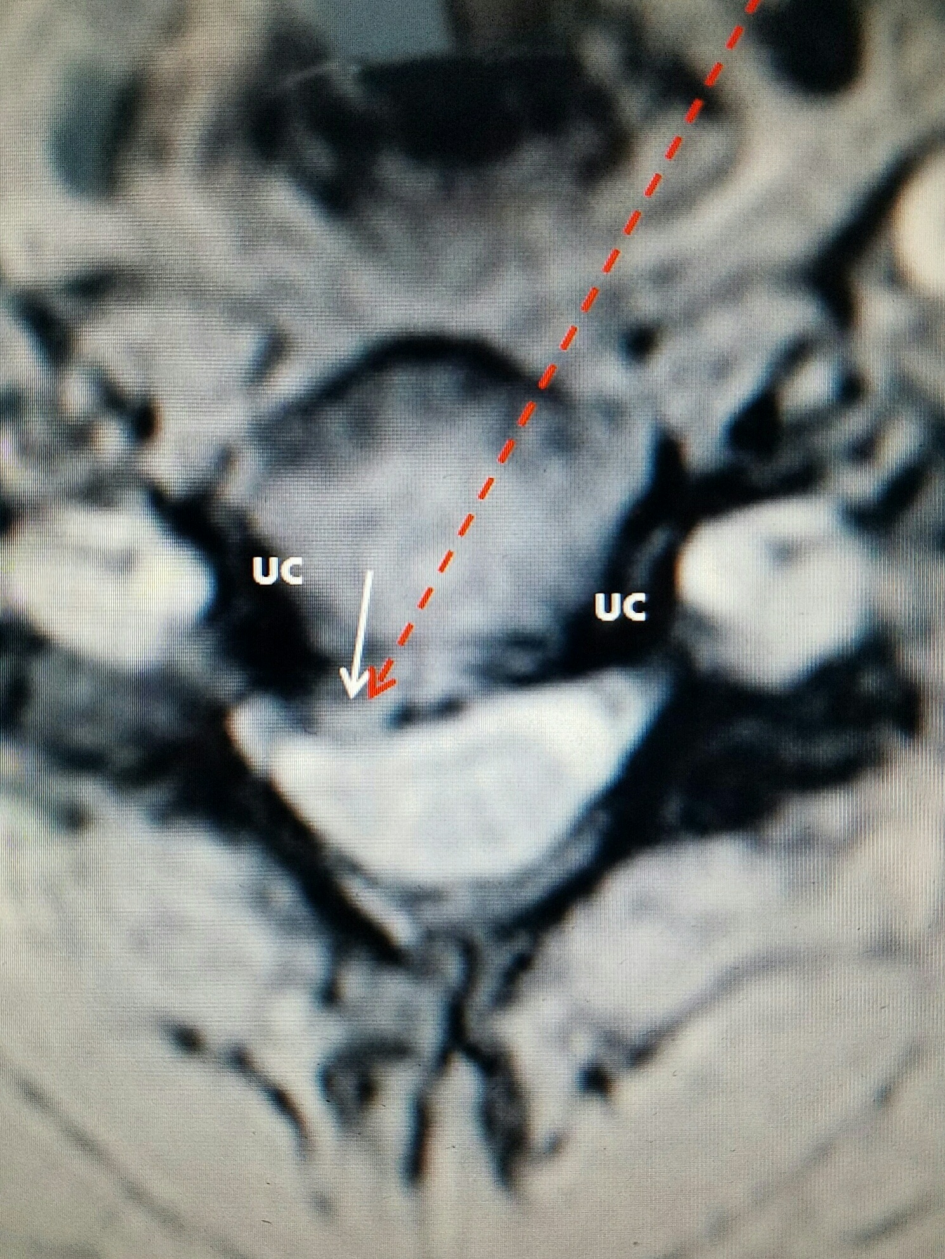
Patient #1: Axial T2 MRI of herniated disc at C3-4 to right The small white arrow shows the right posterolateral disc herniation. The darker lateral uncovertebral joints (UC) appear normal and keep the discectomy instruments confined within the central and paracentral regions of the disc space. The dashed red arrow is the percutaneous path for the access cannula to enter the disc. It is positioned to move across the disc space to the herniation. MRI: Magnetic resonance imaging.
